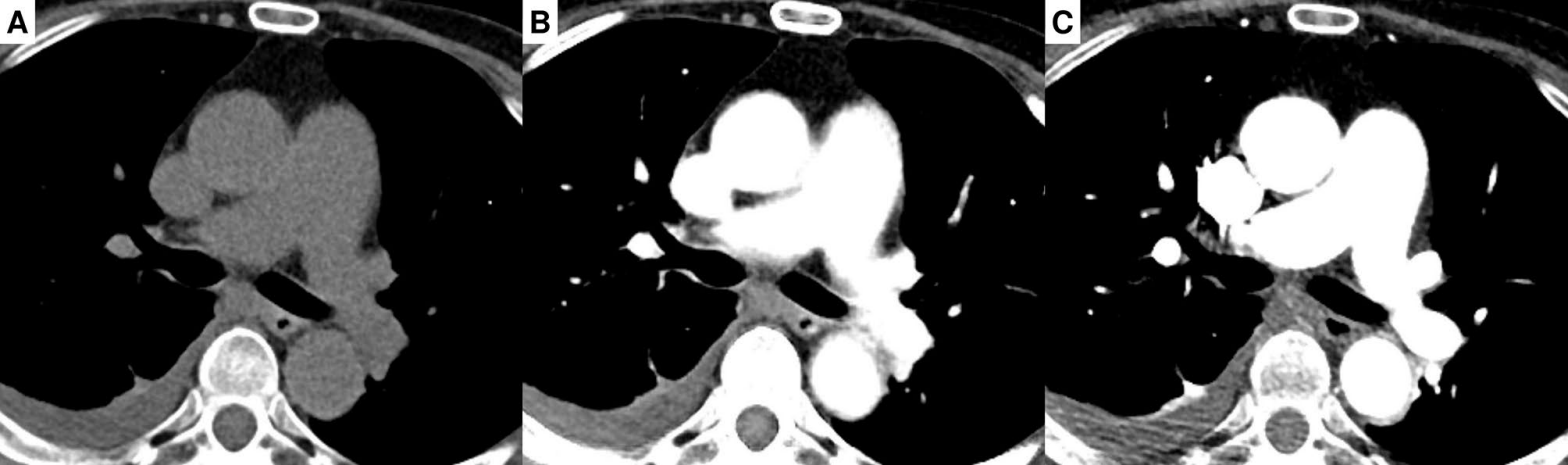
Images of a 65-year-old woman with right pleural effusion from test set 1 are presented. Non-contrast CT (A), synthetic contrast-enhanced CT (B), and contrast-enhanced CT (C).
